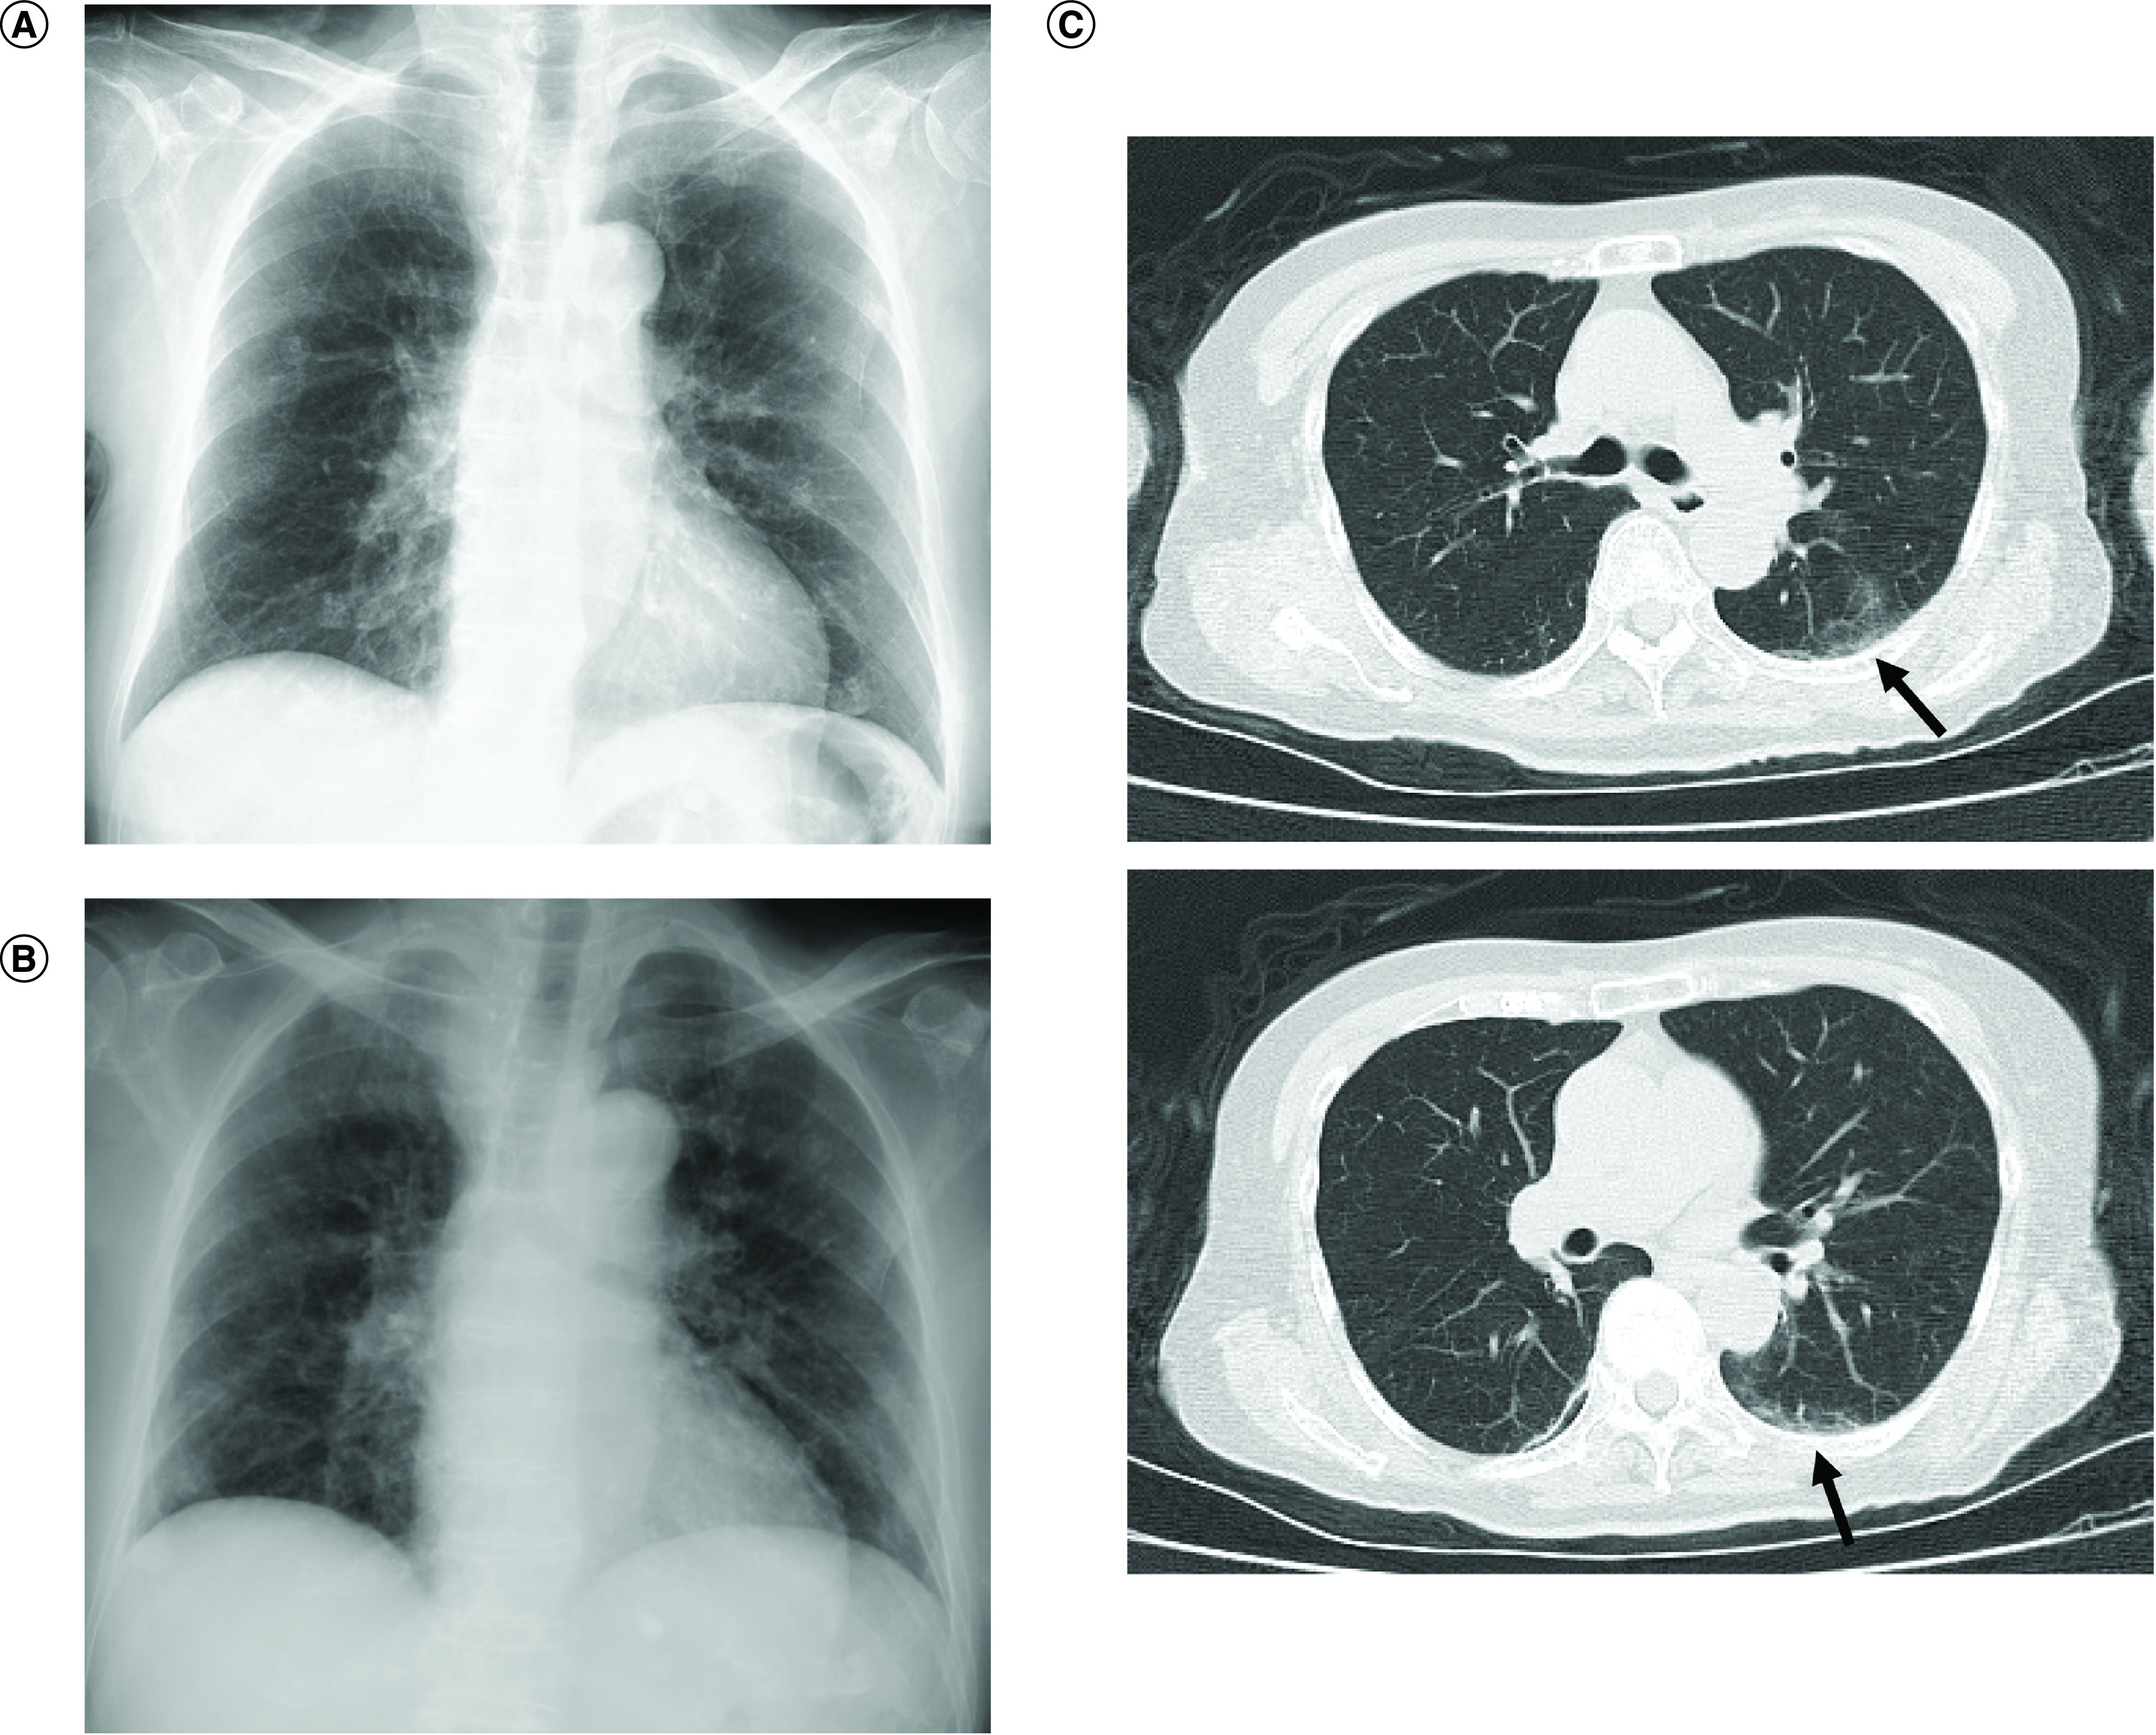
Chest x-ray and computed tomography. (A) Plain chest radiograph at the erect position on day 1. (B) Chest radiograph in the supine position on day 27. (C) Chest computed tomography on day 1. The black arrows indicate ground-glass opacities, suggesting interstitial pneumonia.
